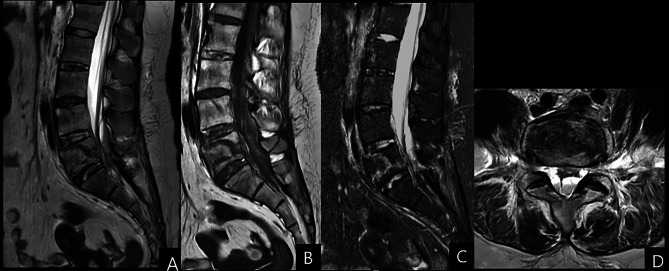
A) T2-weighted sagittal, B) T1-weighted sagittal, C) fat-suppressed sagittal and D) T2-weighted axial MR images show epidural hematoma extending from L4 to S1 in the posterior part of epidural space
